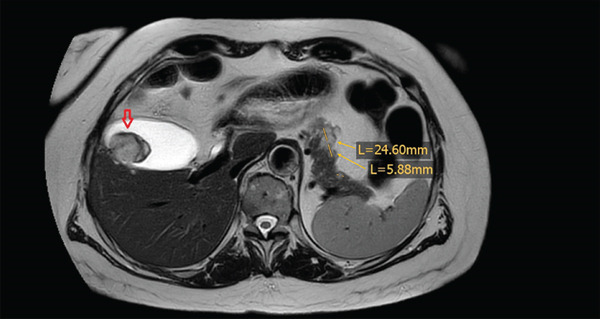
Abdominal MRI showing metastases in the gall bladder (red arrow) and pancreas (orange arrow).
